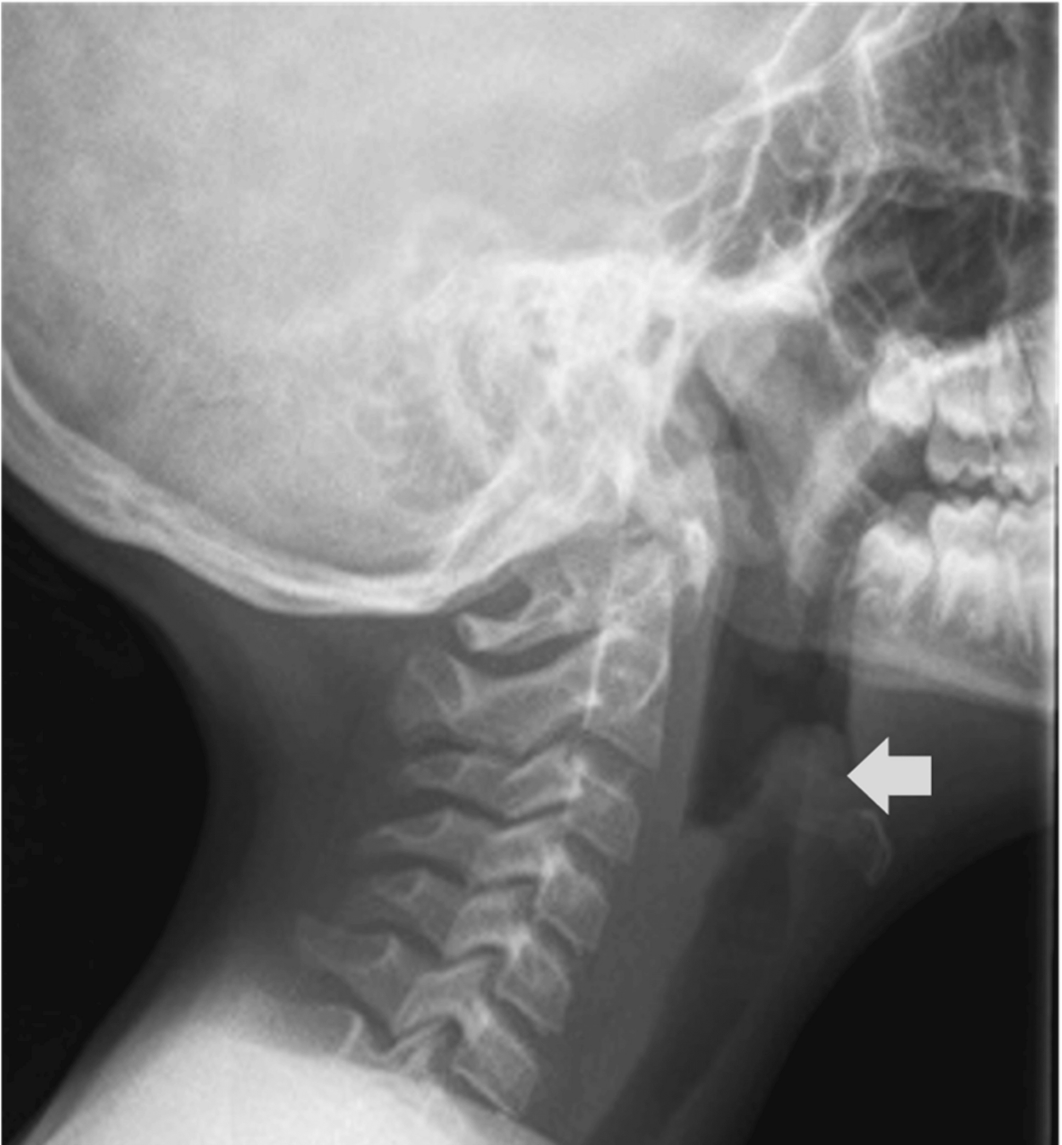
Lateral neck radiograph demonstrating the thumbprint sign The enlarged, edematous epiglottis (white arrow) produces a characteristic thumbprint appearance, consistent with acute epiglottitis.
